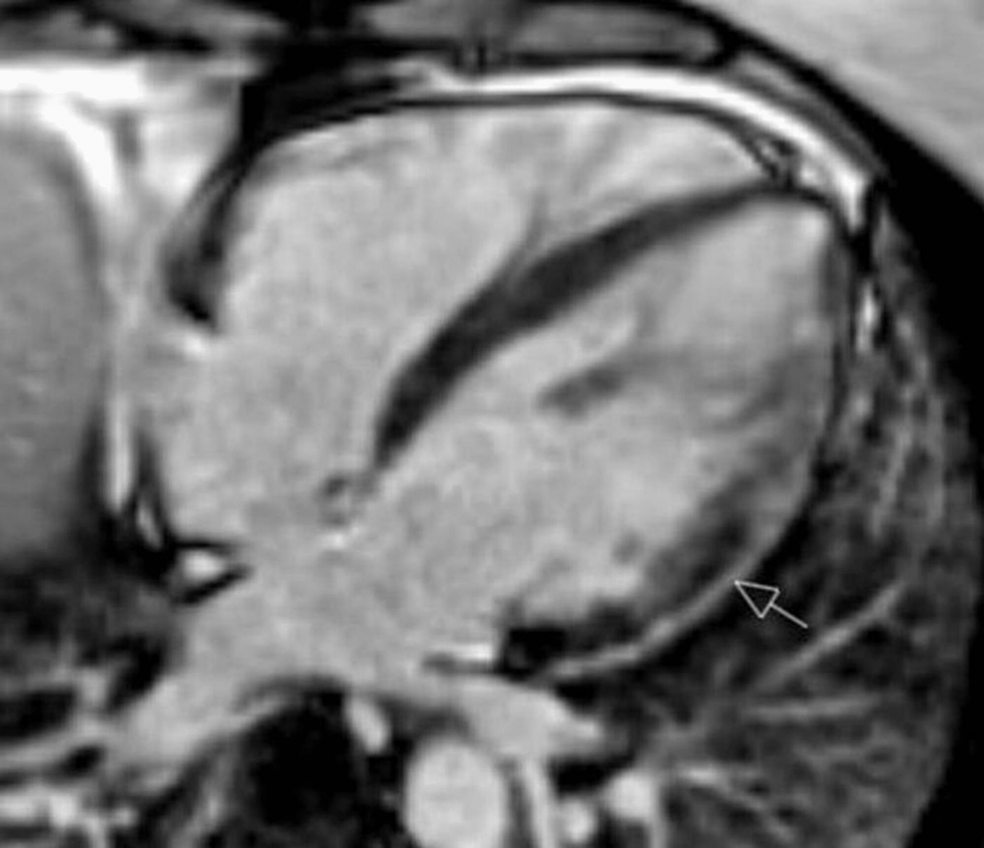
Cardiovascular magnetic resonance imaging showing extensive crescentic-shaped subepicardial late gadolinium enhancement as shown by the arrow.
